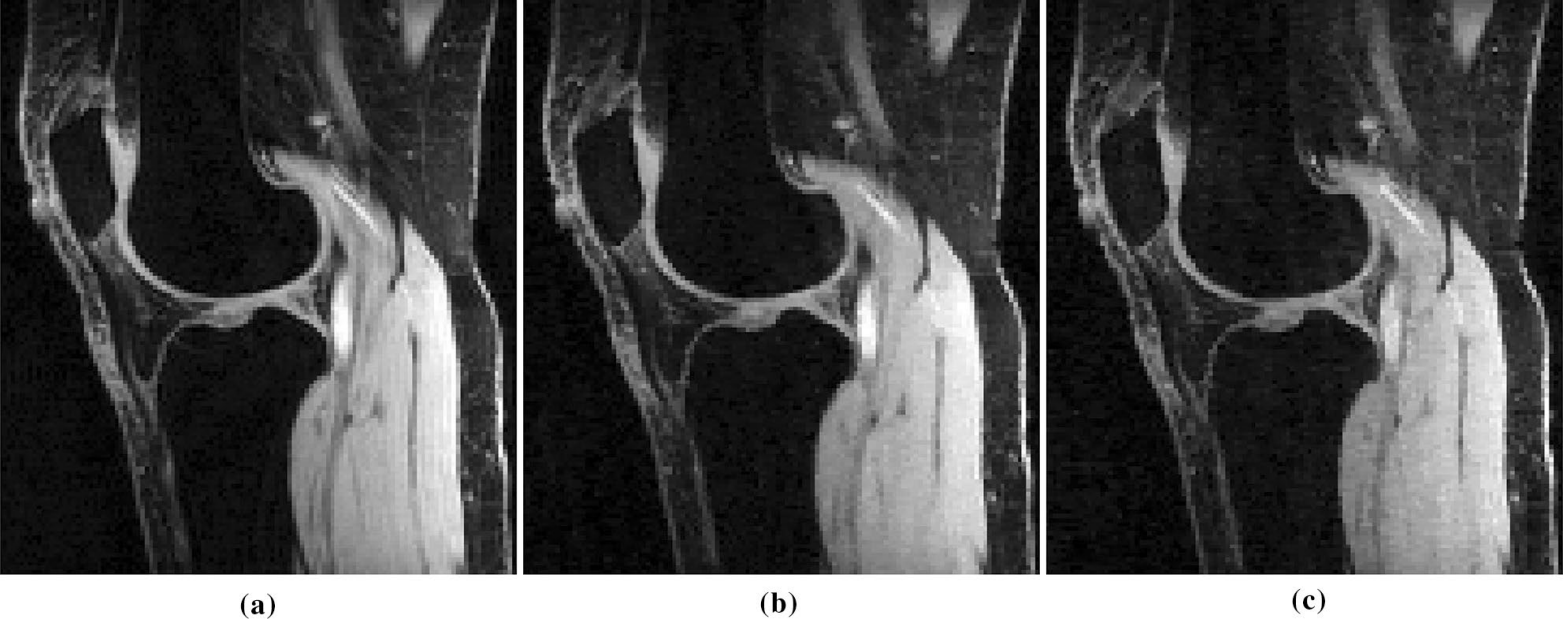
Comparison of (a) fully sampled parallel MRI (2 min 46 s scan time) with prospective accelerated parallel MRI at $$\hbox {AF}=4$$ (42 s scan time) using (b) optimized SP and (c) Poisson disk SP.
